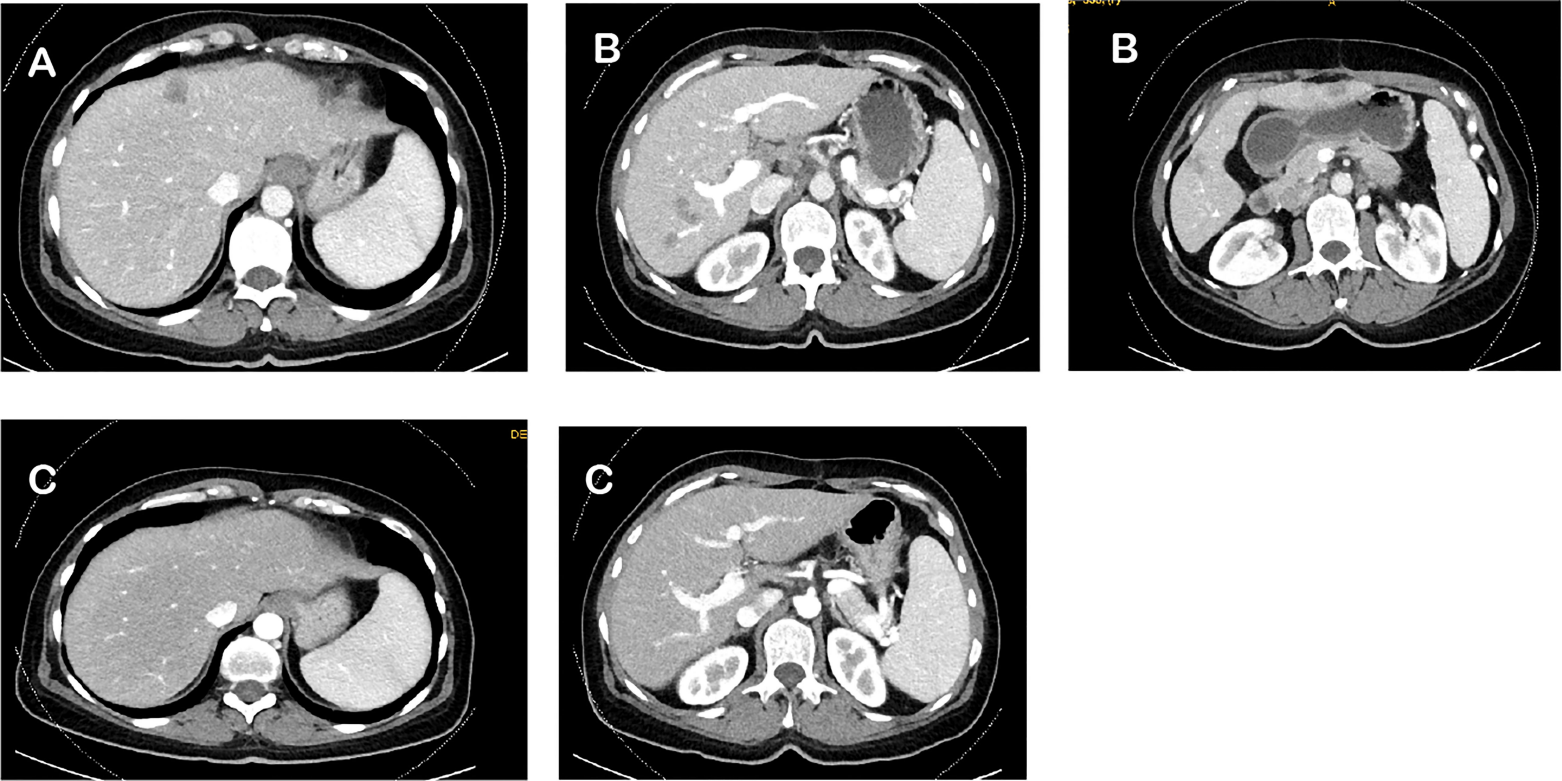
Computed tomography scan (CT scan) regarding the clinical response during whole course of treatment. (A) Baseline CT scan in February 2017. (B) CT scan after adjuvant CAPOX in June 2017. (C) CT scan after 1 year of dabrafenib, trametinib, and cetuximab in October 2018.
